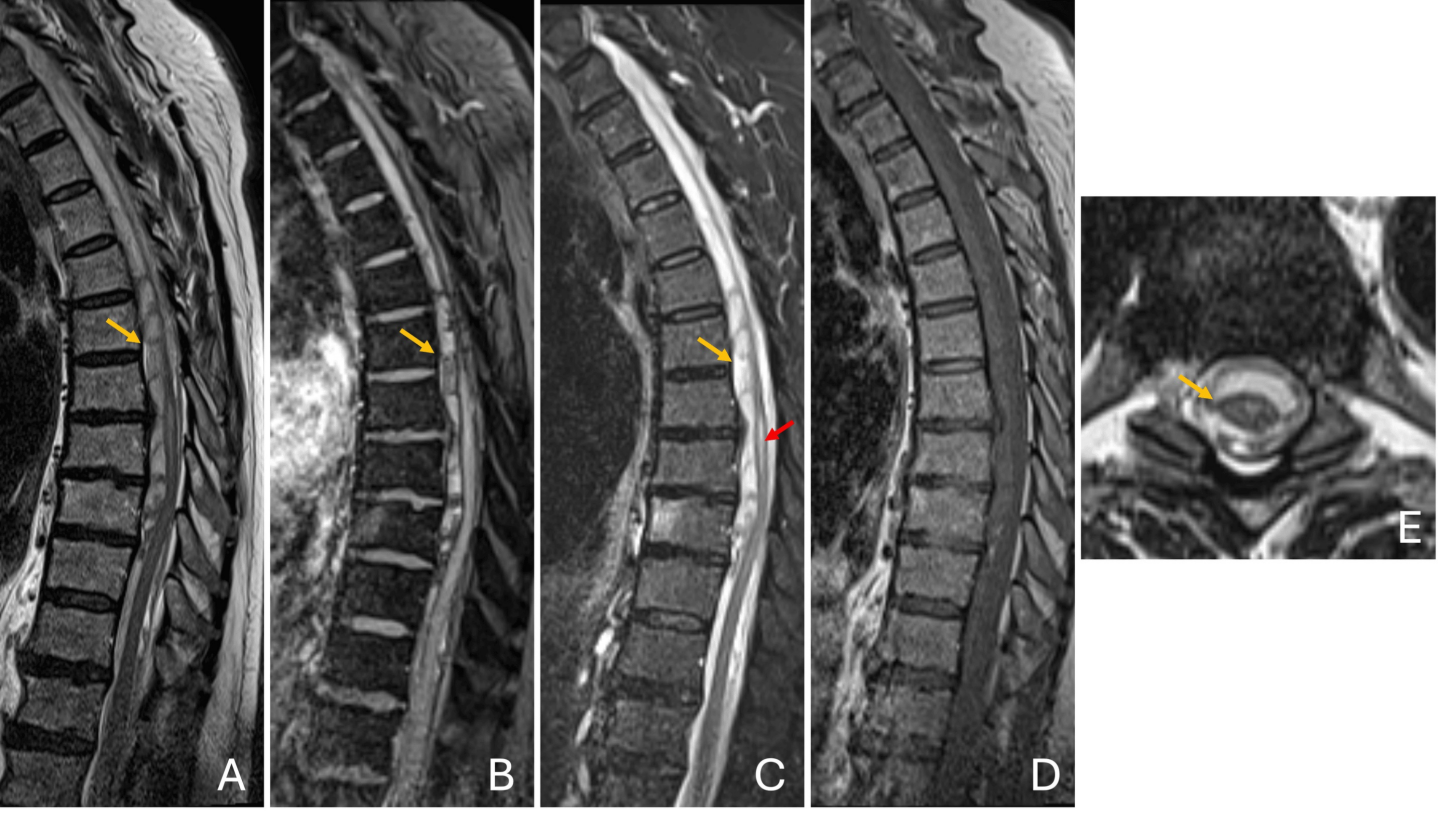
Dorsal MRI. Maximal thickness and septations were observed between T6 and T12 (yellow arrow), as demonstrated on T1-weighted imaging (A), T2* (B), and STIR (C), reaching 8 mm at the level of T8. The hematoma extended caudally to T12, causing posterior displacement and compression of the spinal cord, with centromedullary hyperintensity from T7 to T12, suggestive of edema (C, red arrow). While predominantly anterior, it also extended posteriorly and laterally, without evident restriction by the denticulate ligaments, supporting a subarachnoid location (E), though a subdural component could not be excluded. No abnormal contrast enhancement was observed on sagittal T1-weighted imaging, disfavoring the presence of vascular anomalies, namely arteriovenous shunts and cavernous malformations (D). MRI, magnetic resonance imaging; STIR, short tau inversion recovery.
